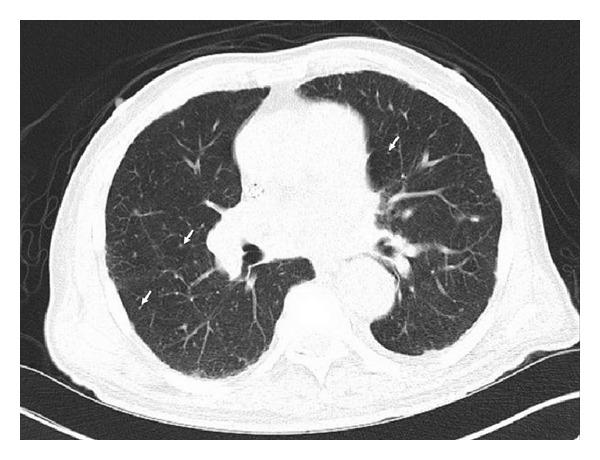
CT scan revealed the diffuse of tiny bilateral pulmonary nodules (arrows) secondary to granulomatous disease particularly with large calcified granulomas with mediastinal and hilar lymphadenopathy. There was evidence of pulmonary arterial hypertension.
